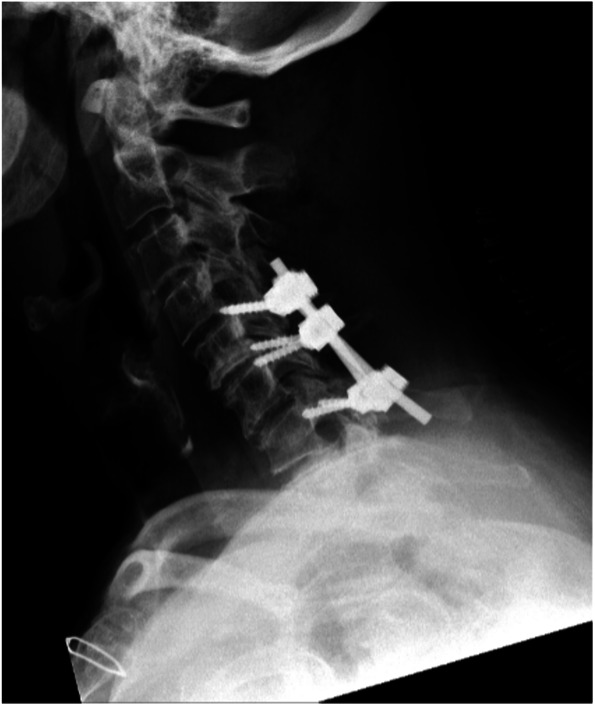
A postoperative x-ray of one of our patients shows posterior lateral mass fixation from C5 to C7. The precise evaluation of the screws' positions is difficult. However, screws seem to be inserted correctly.
